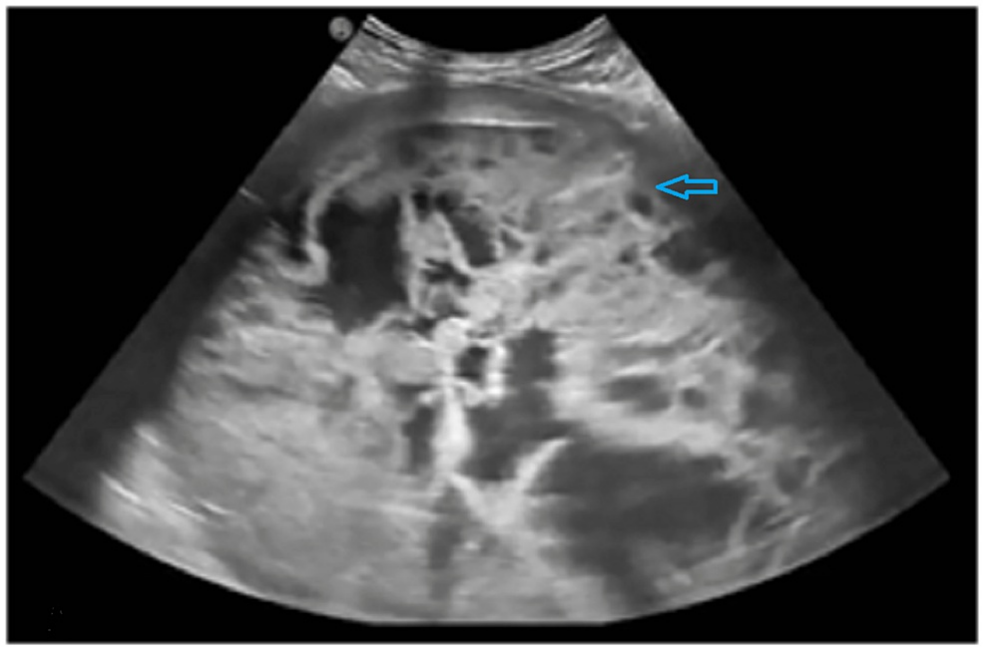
Abdominal ultrasound image Ultrasound showed a well-defined, heterogeneous, rounded abdominal mass, with solid and cystic components.
